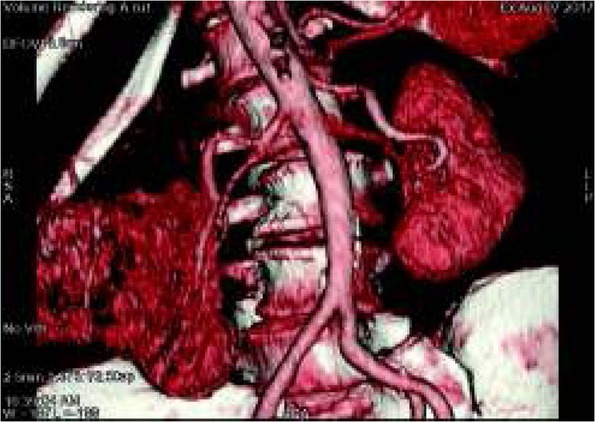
Abdominal enhanced CT. Volumetric reconstruction. Right kidney cancer. Ryc. 8. Badanie CT jamy brzusznej po wzmocnieniu kontrastem, rekonstrukcja objętościowa. Rak nerki prawej.
